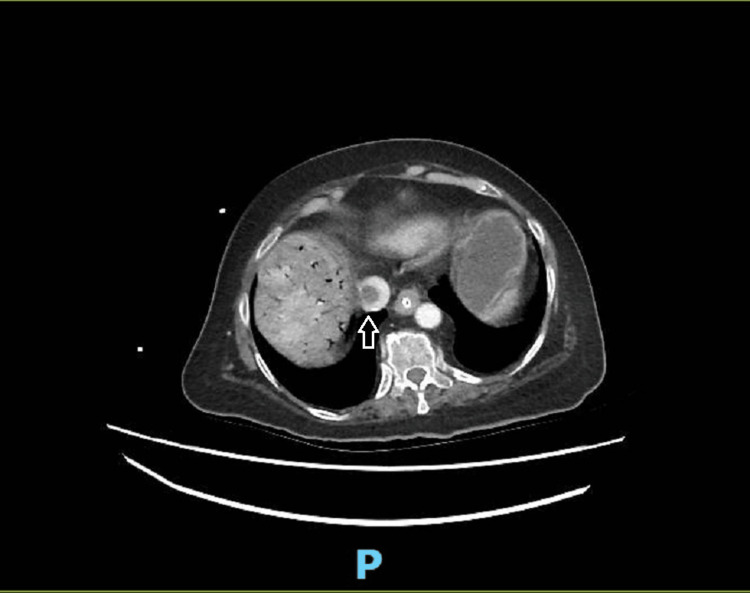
Contrast-enhanced CT (sagittal view) in the portal venous phase on day of admission showing thrombus in the inferior vena cava (IVC).
